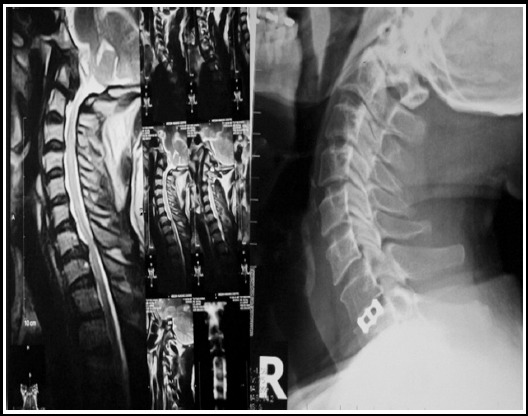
Preop MRI showing c7/T1 prolapse disc(Right) post op x-ray showing Titanium cage(Left).
